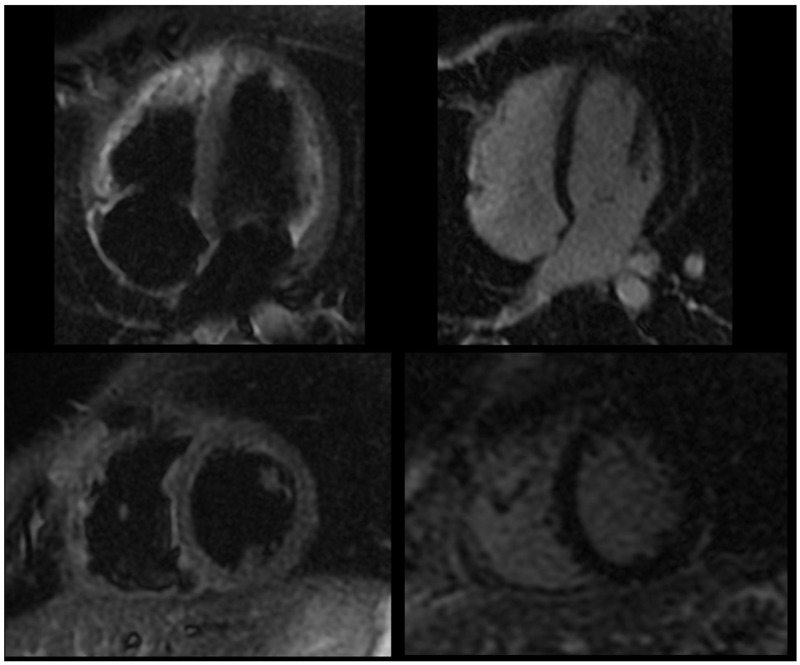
Follow-up cardiac magnetic resonance following anti-IL-1 treatment, showing dark signal in T2-weighted black blood STIR sequences (left panel) and no significant delayed pericardial enhancement (right panel).
